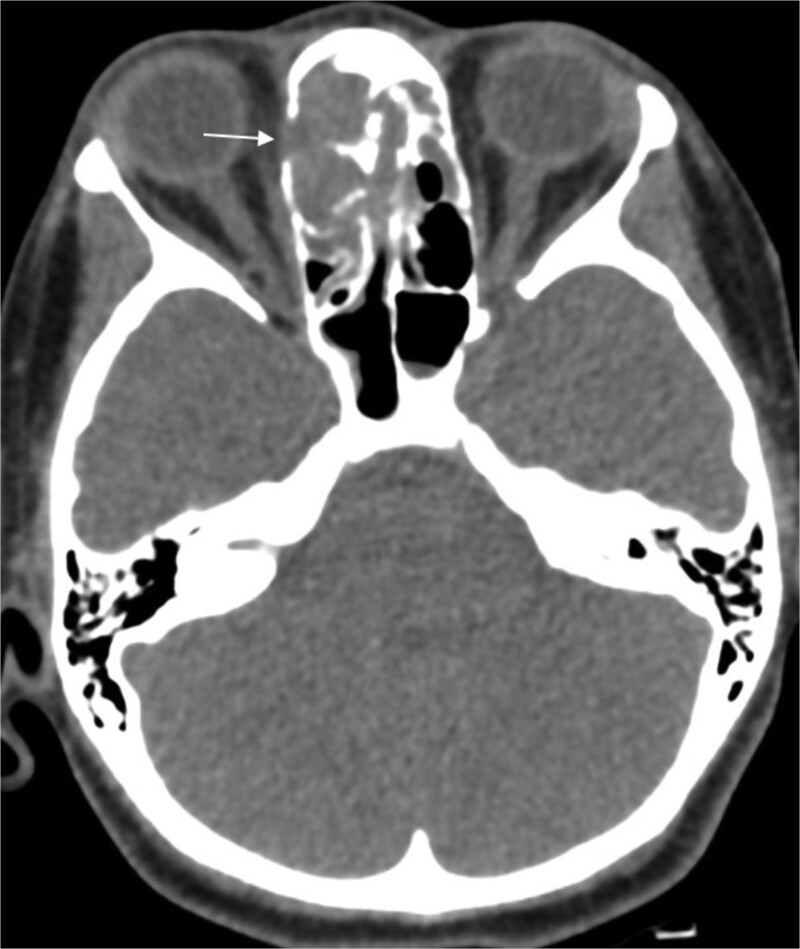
Paranasal CT scan without contrast a mass in the left ethmoid cavity with erosion of the lamina papyracea (arrow).
